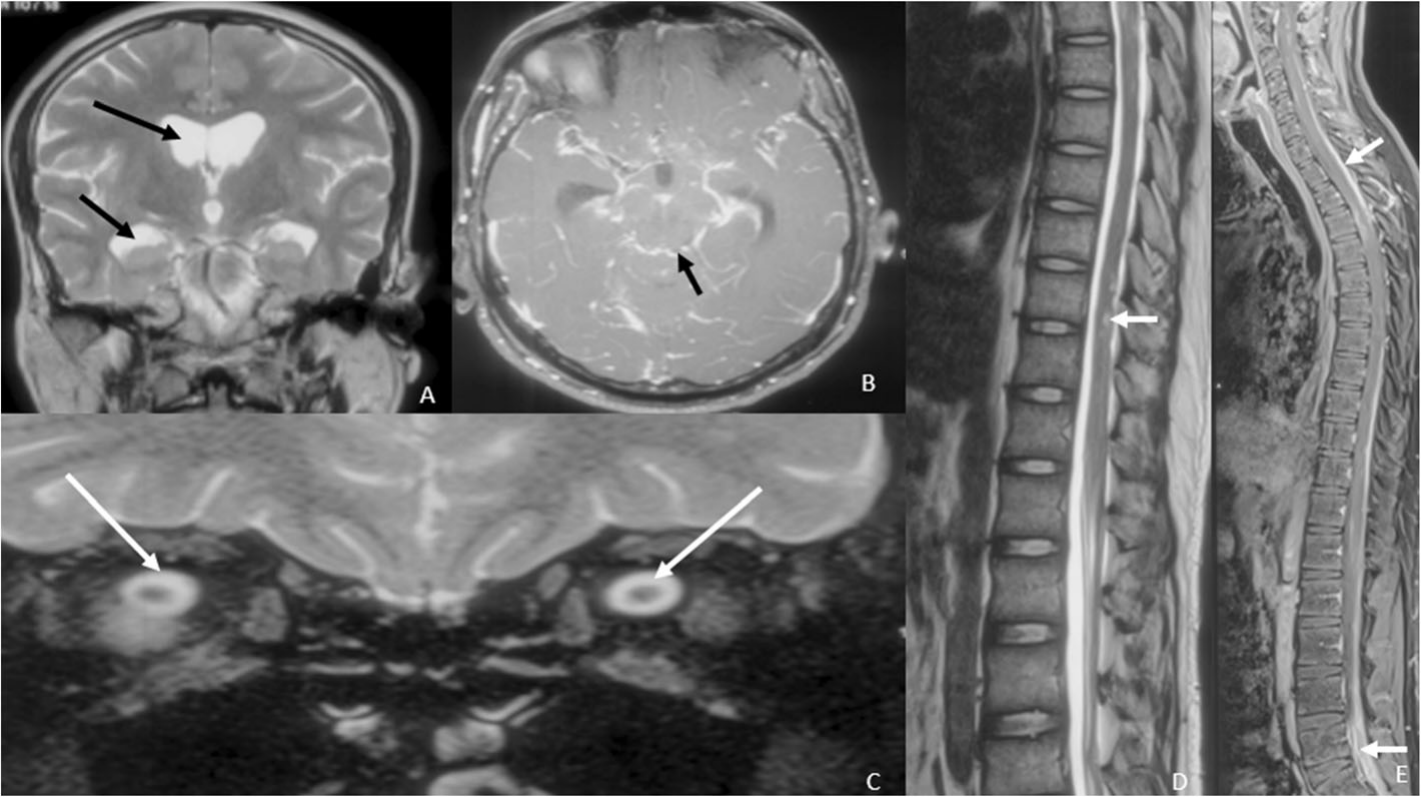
Magnetic resonance imaging of the brain and dorsal spine at 2 months following the onset of symptoms. A T2W coronal image of the brain at the level of the foramen of Monro shows dilated bilateral lateral ventricles. The black arrows point to the dilated frontal and temporal horn of the right lateral ventricle. B T1W post-contrast image of the brain at the level of the mid-brain shows diffuse and thin leptomeningeal enhancement. The black arrow is pointing towards the leptomeningeal enhancement along the quadrigeminal cistern. C T2W coronal image of the brain at the level of the orbits (zoomed to demonstrate optic nerves) shows distended per-optic CSF spaces. The white arrows point towards the distended peri-optic CSF spaces. D Sagittal T2W MRI of the dorsal spine shows few cysts along the dorsal spinal cord. The white arrows point towards the intramedullary cysts within the dorsal cord. E Post-contrast T1W sagittal image of the spine. There is diffuse leptomeningeal enhancement noted along the cord surface and the nerve roots of cauda equina. The white arrows point towards the leptomeningeal enhancement along the dorsal cord and also the cauda equina
